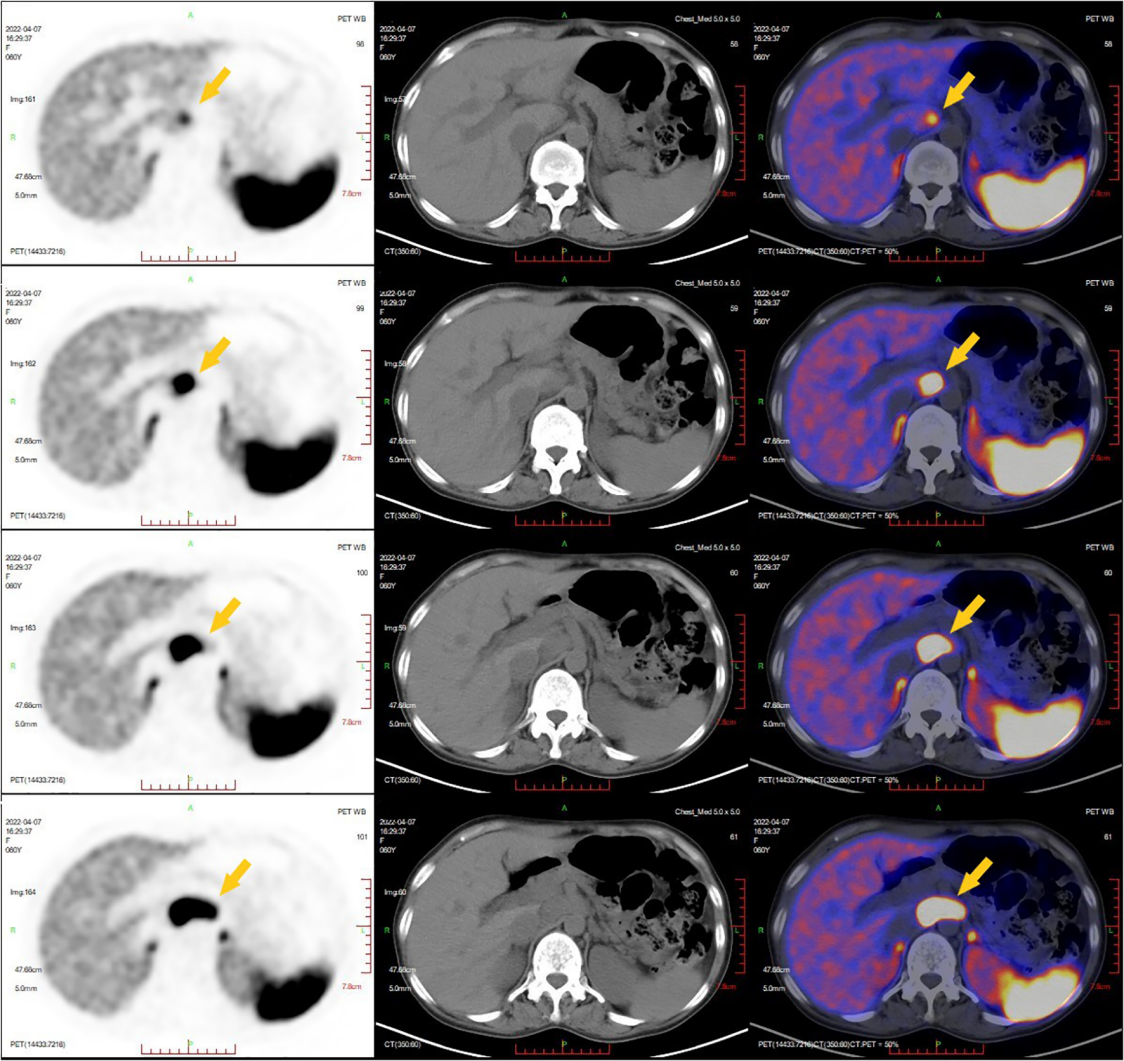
Somatostatin receptor imaging (68Ga PET-CT). The yellow arrow indicated a retroperitoneal mass with abnormal increased expression of somatostatin receptor was consistent with the appearance of paraganglioma.
